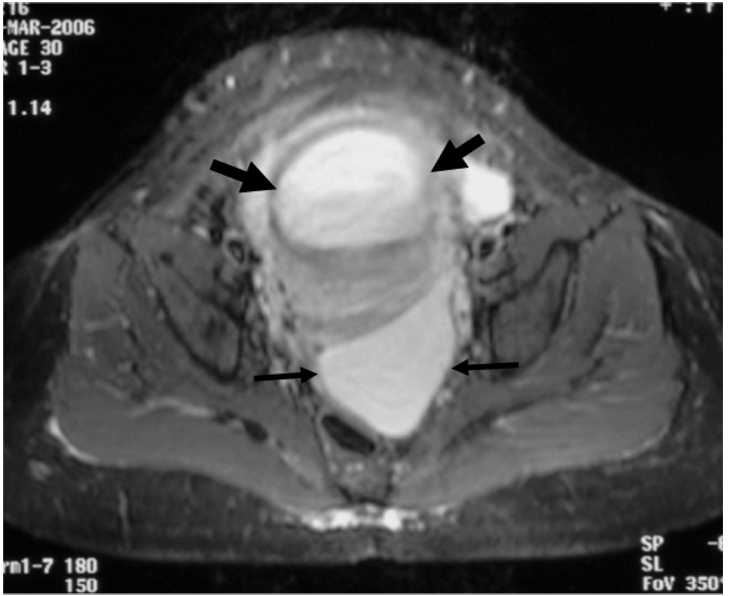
Axial STIR MR image shows a well-defined cystic mass (thin arrows) lying posterior to the gravid uterus (thick arrows).
